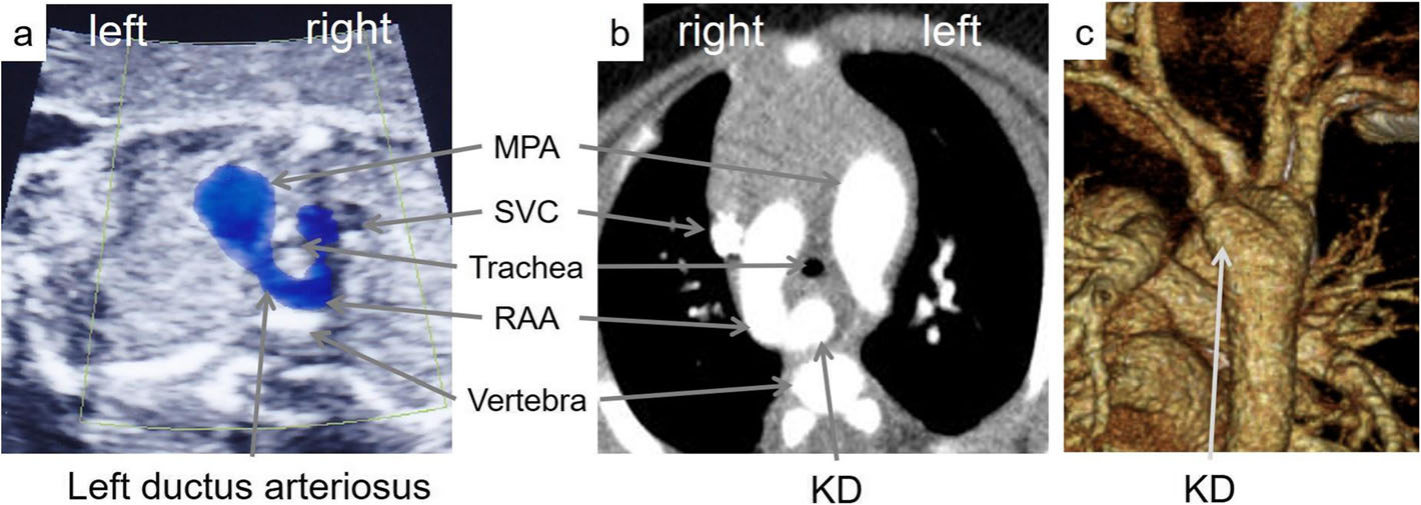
Prenatal ultrasonography and chest CT at preoperative status describing the RAA, retroesophageal LSCA, and KD leading to tracheal compression. a Prenatal ultrasonography from the upper mediastinum. b Horizontal image in chest CT. c 3D image from the left back in chest CT. KD: Kommerell’s diverticulum, LSCA: left subclavian artery, MPA: main pulmonary artery, RAA: right aortic arch, SVC: superior vena cava
